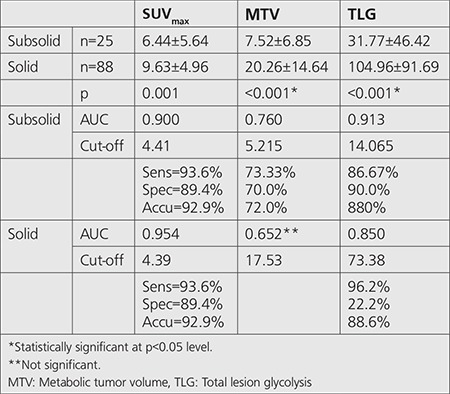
Cut-off values of SUV_max_, metabolic tumor volume, total lesion glycolysis for subsolid and solid solitary pulmonary nodules
